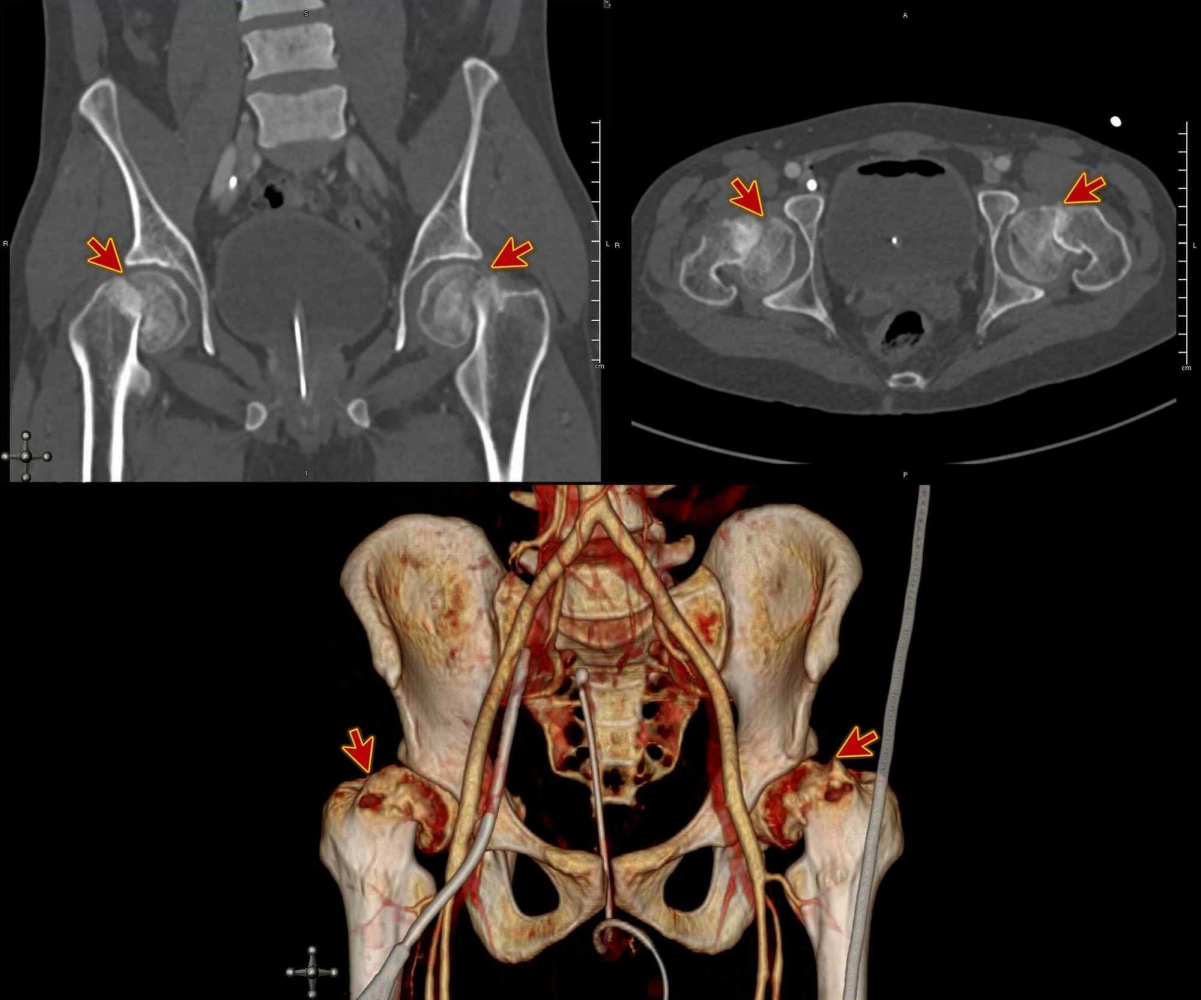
Pelvic CT showing bilateral displaced femoral neck fractures.
